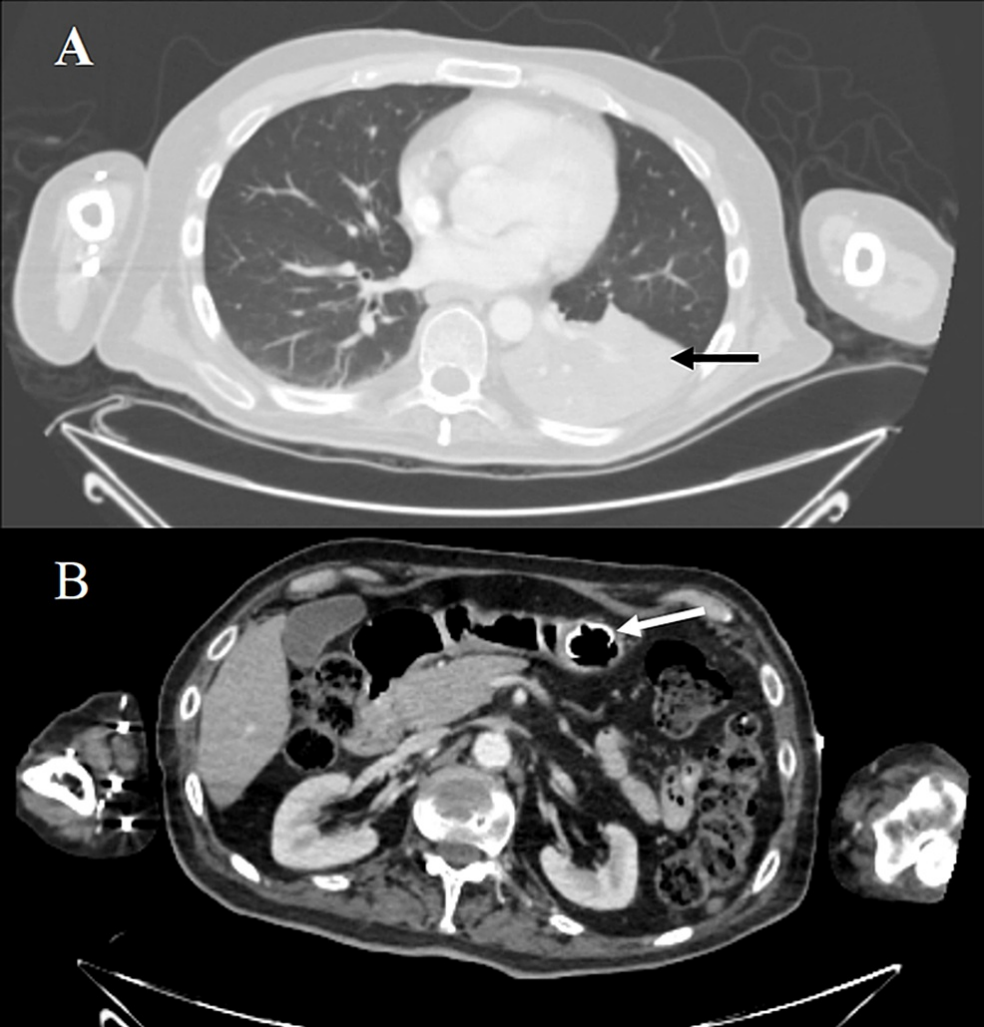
Chest and abdominal CT A. Chest CT showing LLL consolidation with obstruction of the accompanying bronchus with fluid content (black arrow). B. Abdominal CT with PEG tube in place with no evidence of leak or free air (white arrow) CT: computed tomography; LLL: left lower lobe; PEG: percutaneous endoscopic gastrostomy
